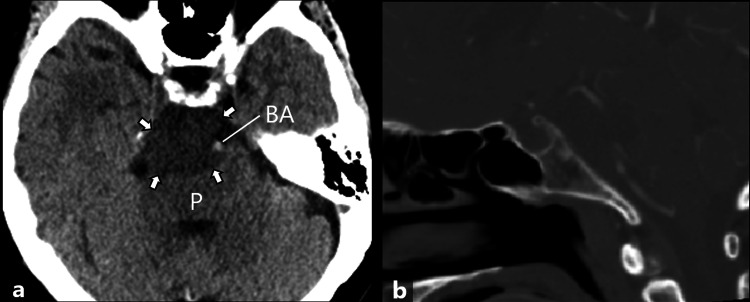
Noncontrast-enhanced head CT of the patient. The images show (a) axial noncontrast-enhanced CT and (b) sagittal image of the contrast-enhanced CT in the bone window. Noncontrast-enhanced head CT shows a 40-mm, well-defined, homogeneous, low-density mass in the prepontine cistern (a: arrows). The pons (P) and basilar artery (BA) are compressed dorsally by the mass. There is no obvious fat or calcification within the mass. No bone destruction of the clivus is seen (b).
